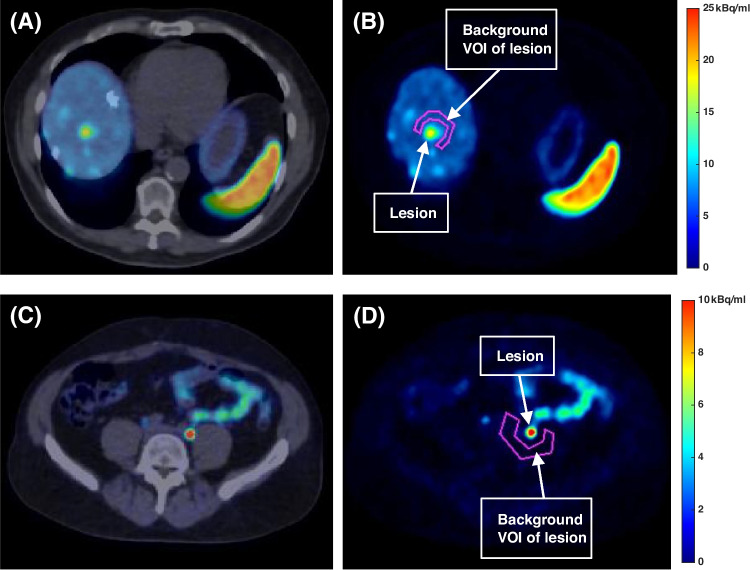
Examples of cross-sections of lesions from the fusion of PET/CT and the corresponding background VOIs. An example of lesion located inside a background with a relatively high activity background concentration (i.e. liver): (A) PET/CT fusion image, (B) background VOI of the lesion in the PET image. Example of lesion with a low background activity concentration: (C) PET/CT fusion image, (D) PET image with the background VOI of the lesion
